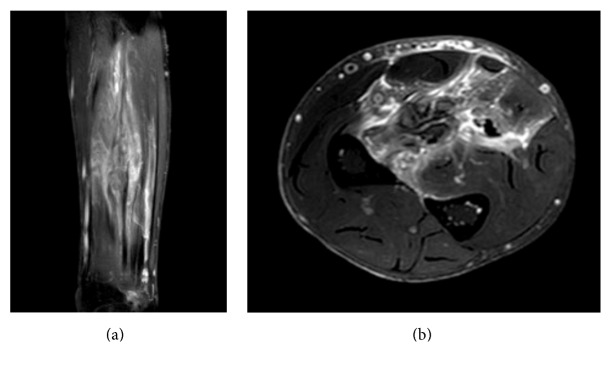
MRI of the right forearm ((a), coronal view; (b), axial view): infiltrating lesion of the superficial and deep volar compartment of the forearm with a widespread edema.
